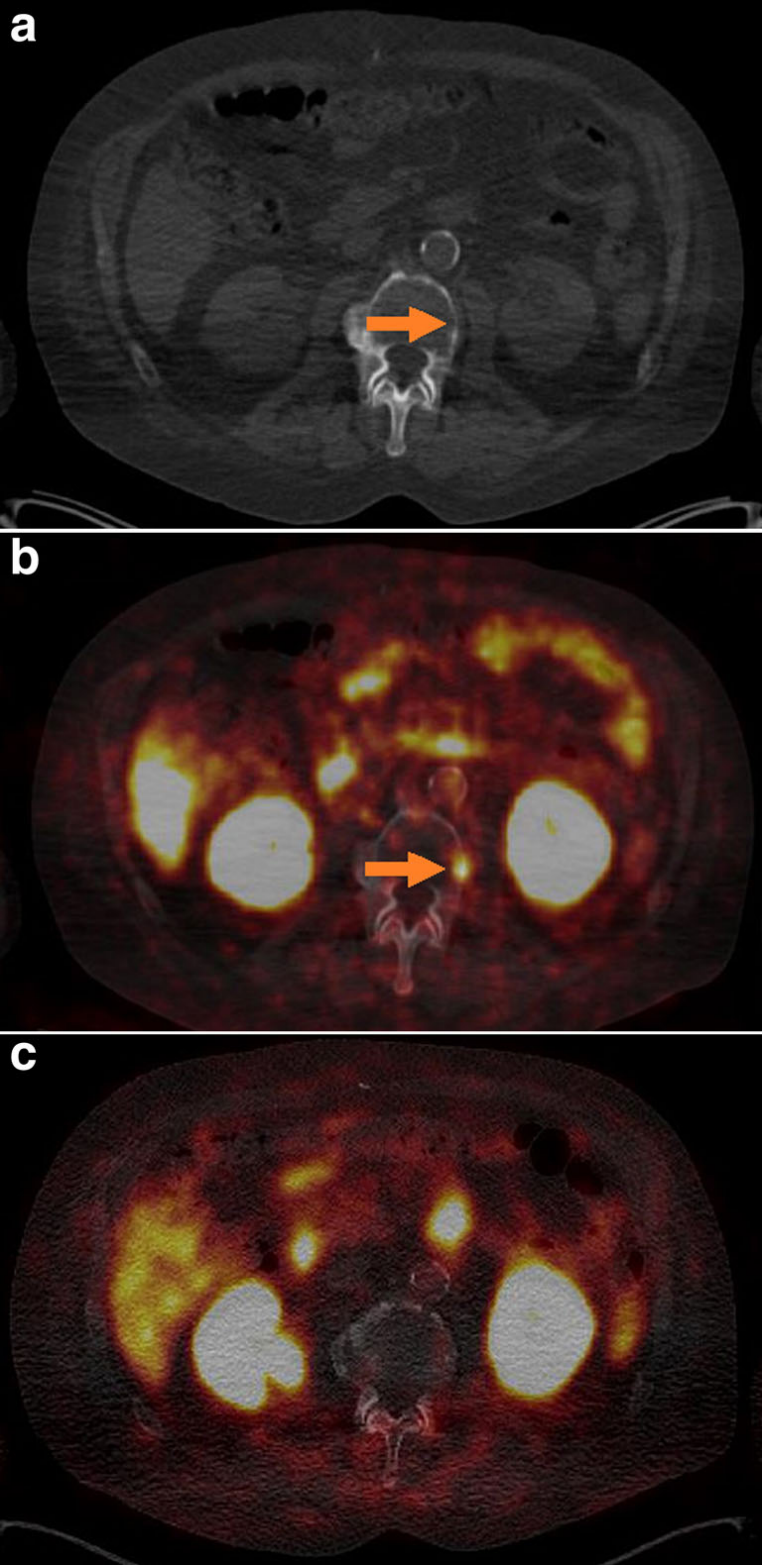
Patient no. 2 with a rising PSA level of 4.7 ng/ml. a Low-dose CT in bone window and b [^18^F]DCFPyL PET/CT were suspicious of bone metastasis in vertebra L2, hard to discern in the c [^68^Ga]Ga-PSMA-HBED-CC PET/CT. Imaging was performed on a Biograph 16 PET/CT.
